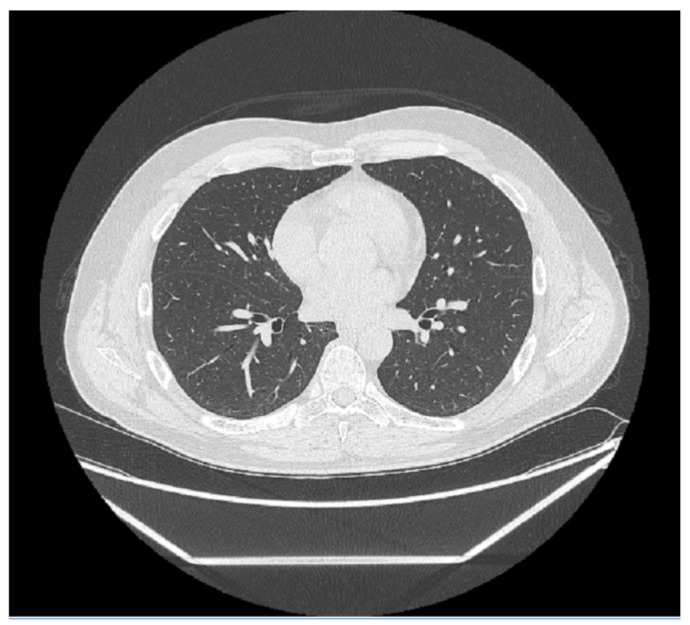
HRCT scan of a healthy patient. The image shows an example of healthy lung parenchyma.
